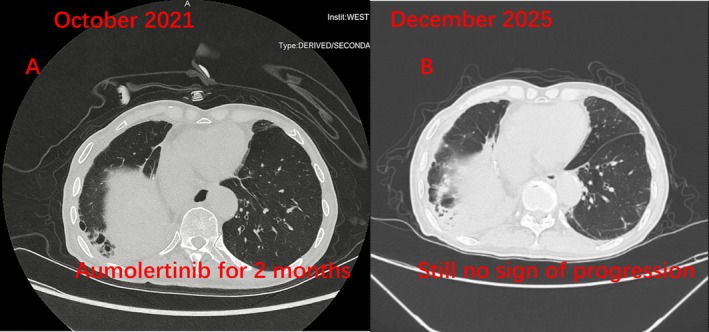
Follow‐up computed tomography images of the octogenarian patient after receiving aumolertinib as second‐line treatment. (A) Computed tomography after taking aumolertinib for 2 months. (B) Computed tomography in December 2025 showing no sign of recurrence.
